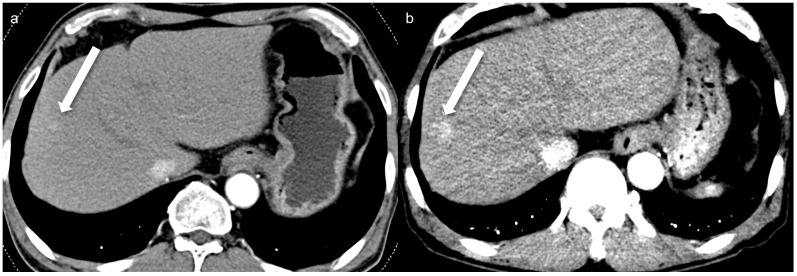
A 67-year-old man with an 8 mm nodular AP shunt. A prior standard-dose CT performed 4 months ago (a) shows subtle arterial enhancing nodular lesion (arrow), whereas a low-concentration contrast medium (270 mg I/mL) monoenergetic 40 keV image (b) shows more prominent arterial enhancement (arrow). The enhanced conspicuity on the monoenergetic image highlights the potential diagnostic benefit of monoenergetic 40 keV imaging in detecting subtle arterioportal shunts.
